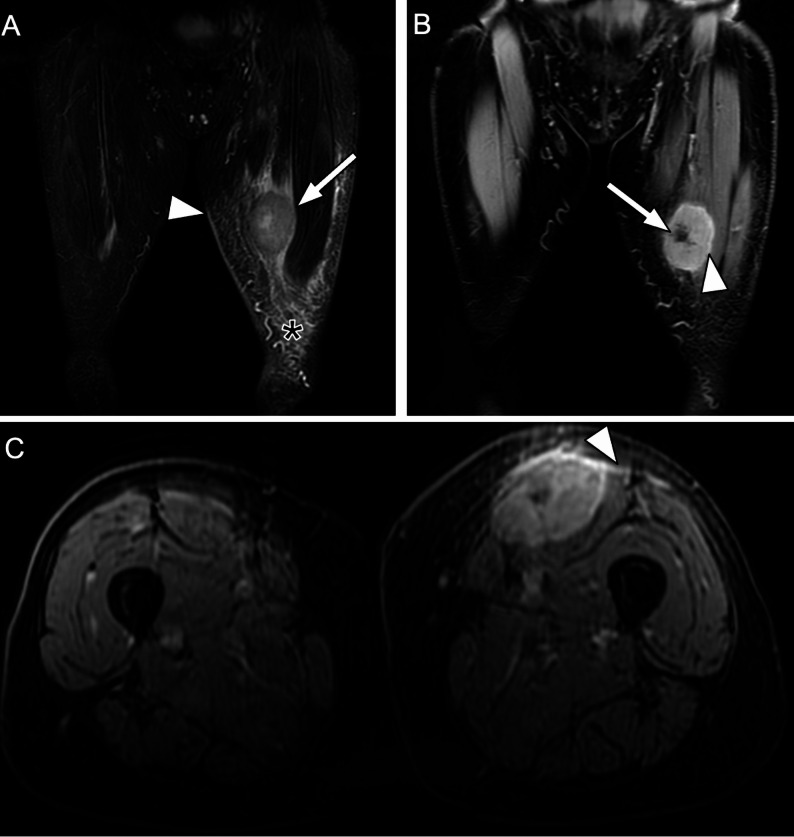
MRI of a 79-year-old female presenting with non-Hodgkin lymphoma manifestation in the left thigh. A Coronal STIR image shows a hyperintense tumor (arrow) with associated enlargement of the affected extremity. Furthermore, subcutaneous stranding (asterisks) and skin thickening (arrowhead) can be noted. B Coronal contrast enhanced fat-saturated T1-weighted image reveals central necrosis (arrow) and peripheral contrast enhancement (arrowhead). C Transversal contrast enhanced fat-saturated T1-weighted image reveals signal enhancement of adjacent fascia lata (arrowhead)
